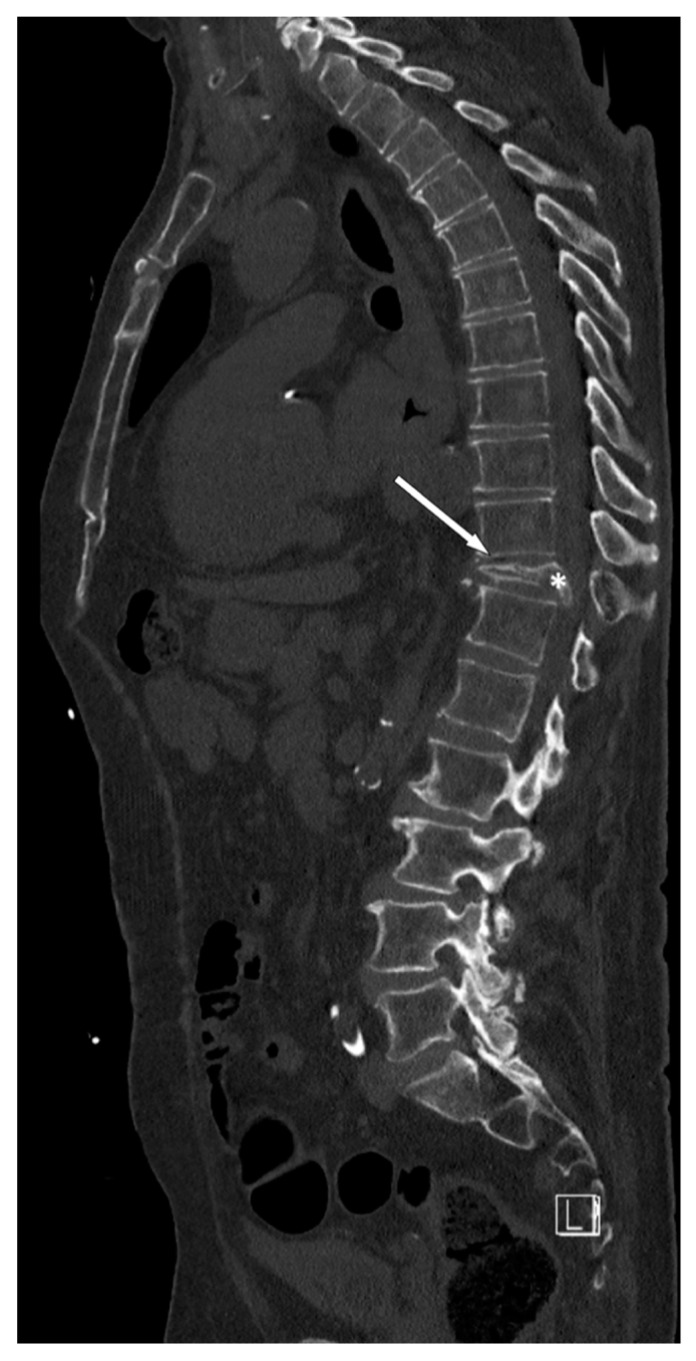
A 70-year-old male with amyotrophic lateral sclerosis was admitted to the emergency department due to progressive respiratory failure and hyponatremia. Sagittal CT scan showing vertebra plana in T11 with neurologic compression from vertebral body collapse (asterisk). A diagnosis of atraumatic chronic osteoporotic fracture is supported by disc degeneration suggested by vacuum disc (white arrow) and bone sclerosis.
